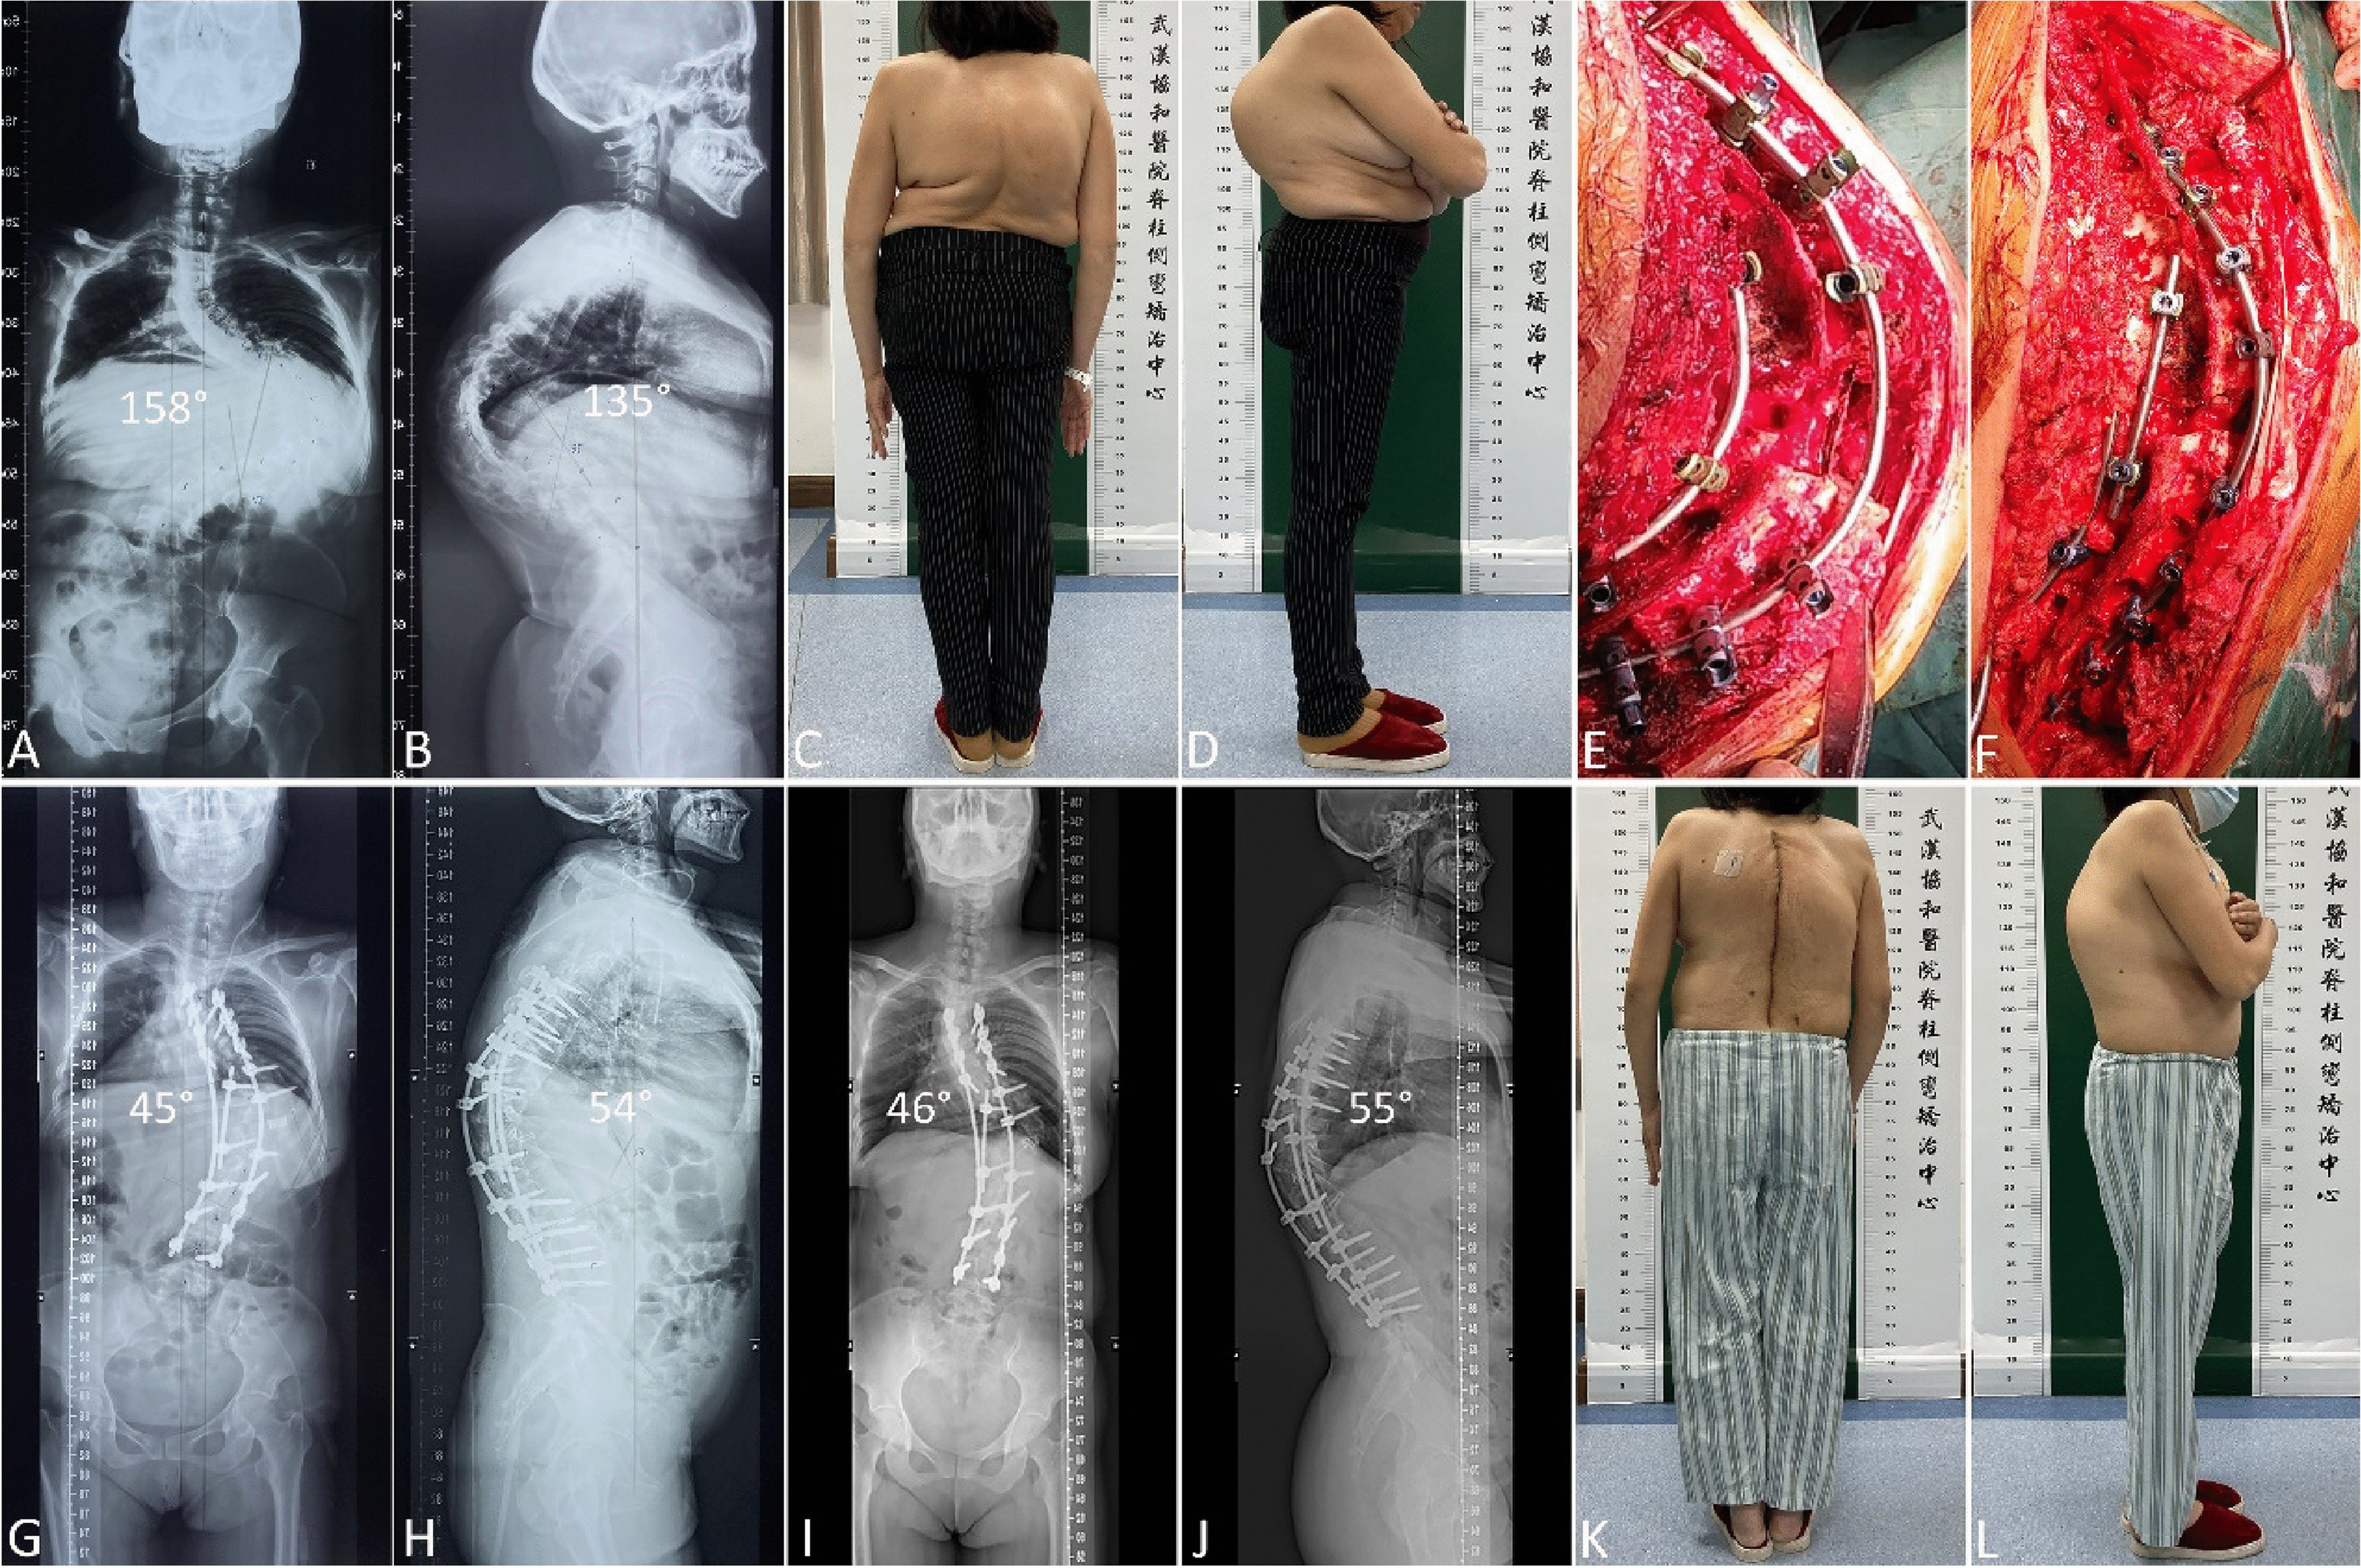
A 48-year-old woman with severe and rigid kyphoscoliosis treated by sequential correction combined with posterior grade 5 spinal osteotomy at the apical vertebrae. A, B, The coronal major curve angle and the kyphotic angle were 158° and 135° respectively on preoperative anteroposterior and lateral radiographs. C, D, Preoperative appearance. E, Grade 5 spinal osteotomy performed at the apical vertebrae (T12), apical vertebrae correction maintained with short segmental instrumentation. F, Sequential correction completed. G, H, The coronal major curve angle and the kyphotic angle were 45° and 54° respectively on postoperative anteroposterior and lateral radiographs. I, J, The coronal major curve angle and the kyphotic angle were 46° and 55° respectively on postoperative anteroposterior and lateral radiographs at 24-month follow-up. K, L, Postoperative appearance
